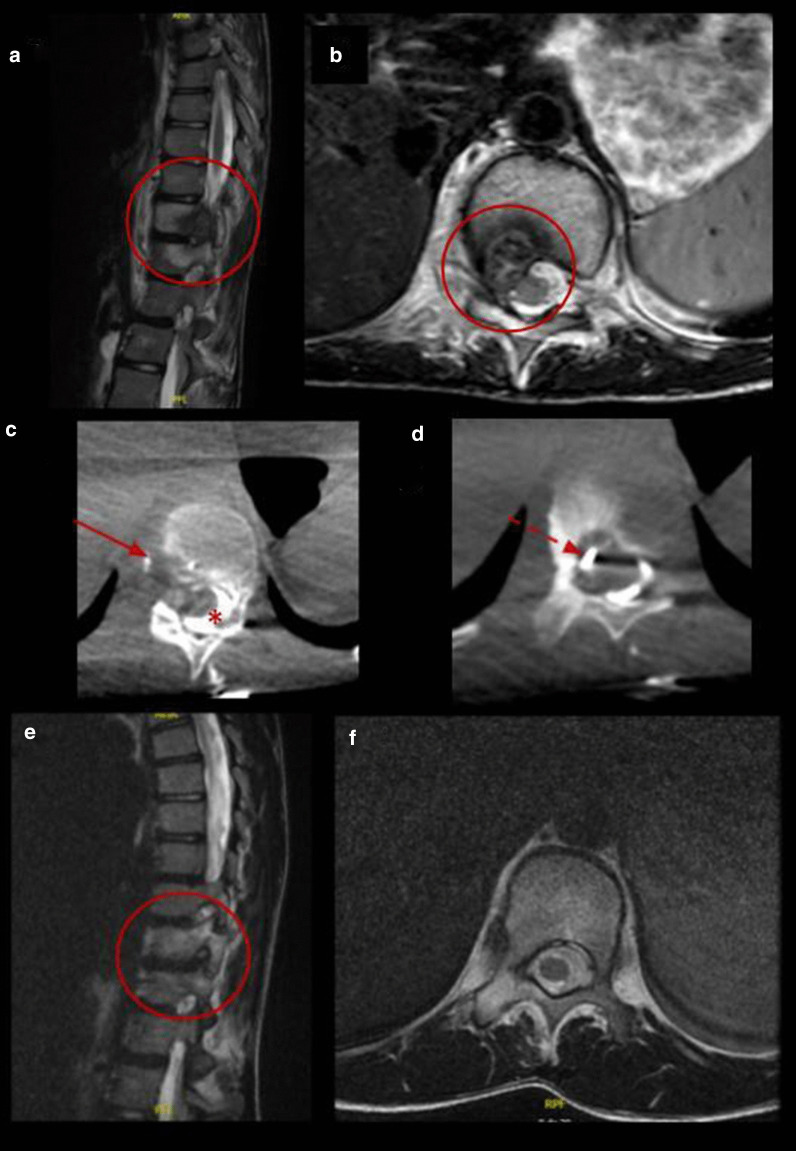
Percutaneous cryoablation of vertebral osteoblastoma in a 14-y.o patient. a, b Sagittal and axial T2 MR images show a lobulated lesion located in the posterior aspect of the body of the 11th thoracic vertebra (red circle). c, d Cryoablation of OB (arrows). Cone beam CT axial reconstruction of the cryoprobe and epidural hydrodissection (asterisk). e, f Follow-up MRI 12 months after procedure with complete resolution of the lesion (red circle)
